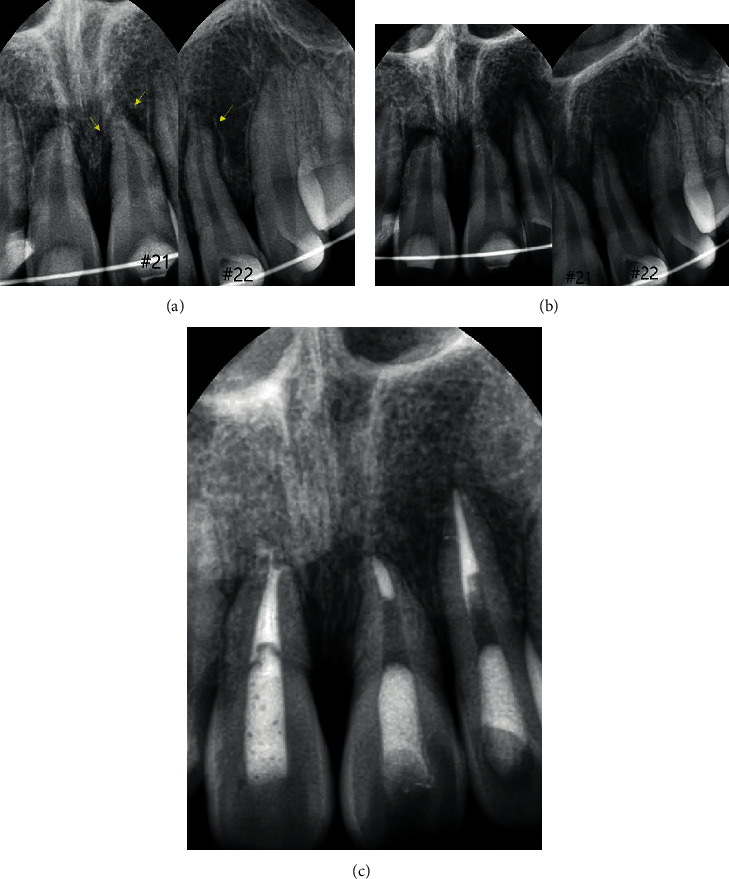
(a) Splinting of the avulsed teeth after replantation. The space around the roots (yellow arrows) indicates improper repositioning. (b) Periapical radiographs two weeks after traumatic injury. (c) Endodontic treatment of the avulsed teeth all completed 14 weeks after the trauma.
